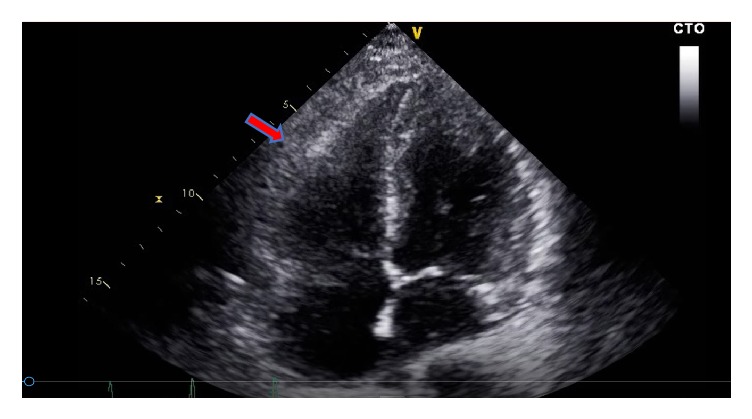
Transthoracic echocardiogram 24 hours after t-PA administration: showing improvement in RV size (arrow) and function but still dilated.
